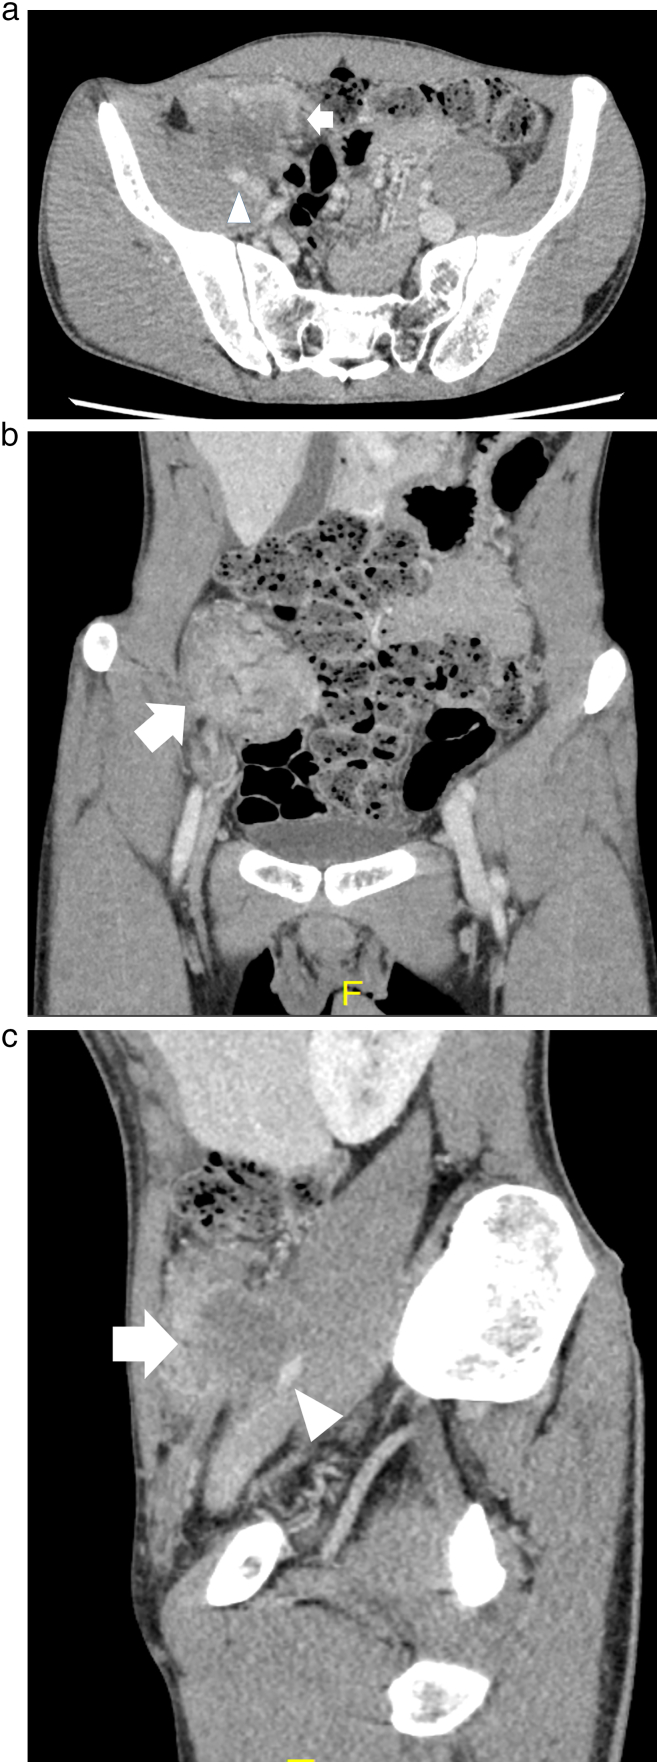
(a) Computed tomography (CT) findings at the first visit (axial). The cecal tumor invading the external iliac artery (arrow) and stenosis of the external iliac artery (arrowhead). (b) CT findings at the first visit (coronal). The cecal tumor (arrow). (c) CT findings at the first visit (sagittal). The cecal tumor invading the external iliac artery (arrow) and stenosis of the external iliac artery (arrowhead).
